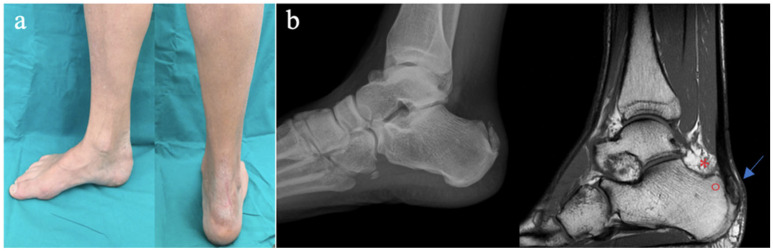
(a) Preoperative clinical images of Haglund’s triad; (b) preoperative radiographical images of Haglund’s triad showing Haglund’s deformity (°), insertional Achilles tendinosis (arrow), or retrocalcaneal bursitis (*).
